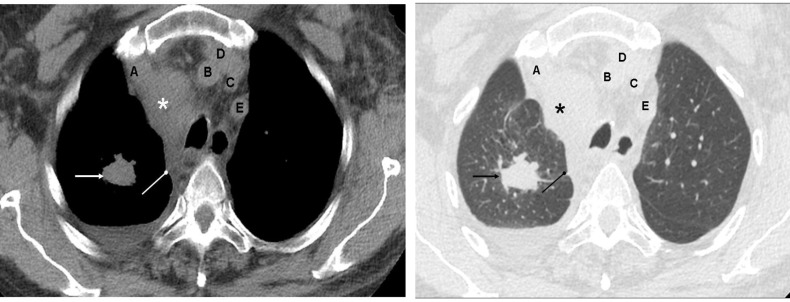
Mediastinal (first image) and lung (second image) windows of a non-contrast CT of the chest of a 55 years old smoker with bronchogenic carcinoma show the primary tumor in the right upper lobe (arrow), enlarged right upper paratracheal (asterik) and retrotracheal (oval arrow) lymph nodes and right sided pleural effusion. Speculated margin of the tumor is better appreciated on the lung window. It is to be noted that pulmonary pathologies (e.g. carcinoma, pneumonia, bronchiectasis etc.) are best assessed on the lung window while extra-pulmonary pathologies (e.g. lymphadenopathy, mediastinal tumor, hematoma, aortic dissection, pulmonary embolism etc.) are best appreciated on the mediastinal window. Vessels are labelled as A to E using same nomenclature as in Figure 1
